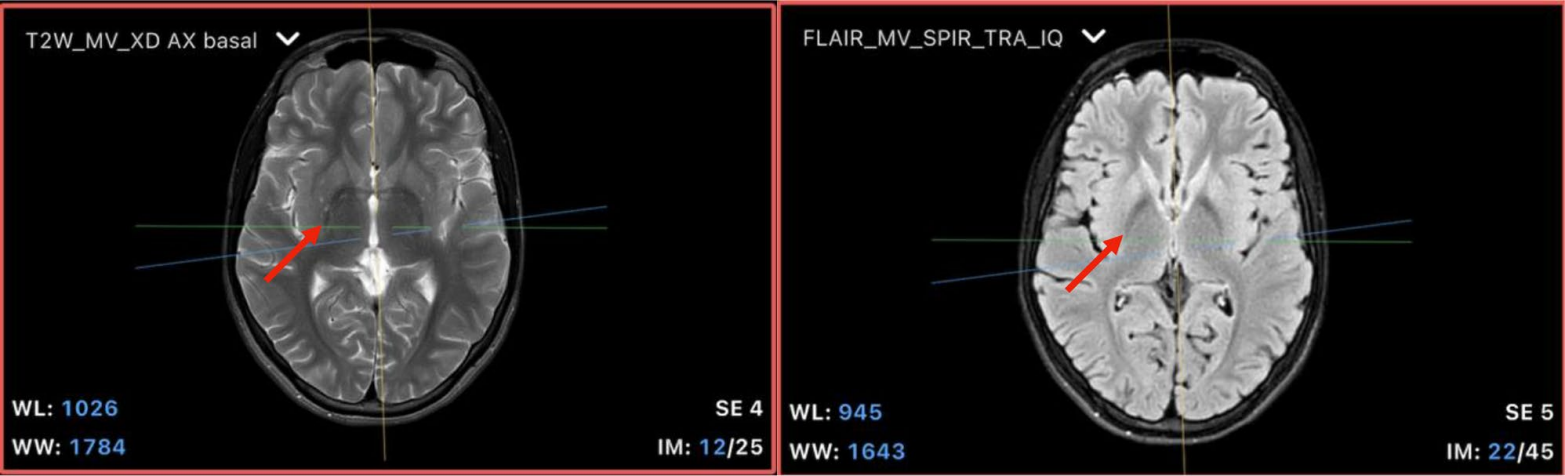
Axial MRI showing iron deposition in the globus pallidus: MRI showing bilateral iron deposition in the globus pallidus, highlighted by susceptibility-weighted imaging (SWI). Left: Axial T2-weighted sequence showing bilateral hypointensity in the globus pallidus (red arrow), suggestive of iron deposition. Right: Axial FLAIR sequence confirming the magnetic susceptibility alteration in the globus pallidus (red arrow), with preservation of the internal medullary lamina
